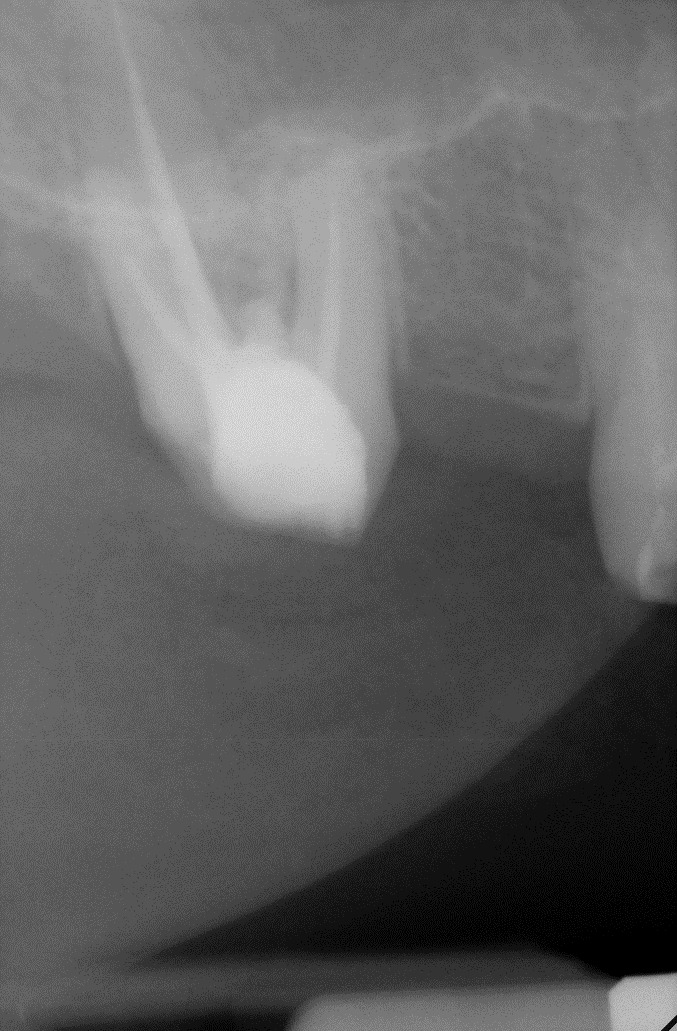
1.6 Retreatment, via falsa has been filled by using MTA; final radiography
